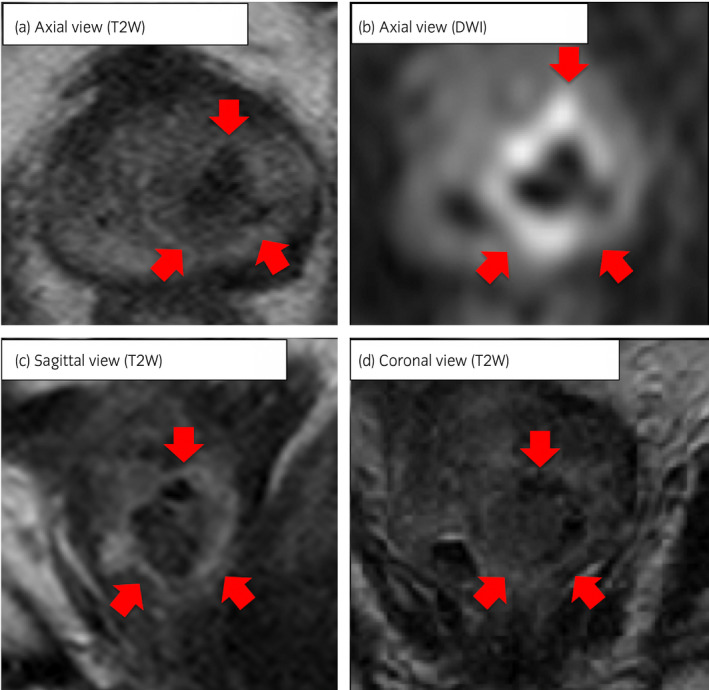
Pelvic magnetic resonance imaging showing an unclear boundary between the prostatic urethra and the transition zone, and the lesion showing signal intensity decreasing from the middle to the apex on T2‐weighted images (a, c, d). The same lesion showing an unclear boundary, and high signal intensity outside the lesion on the diffusion‐weighted image (b). Arrows showing an unclear boundary contour of the prostate SCC.
